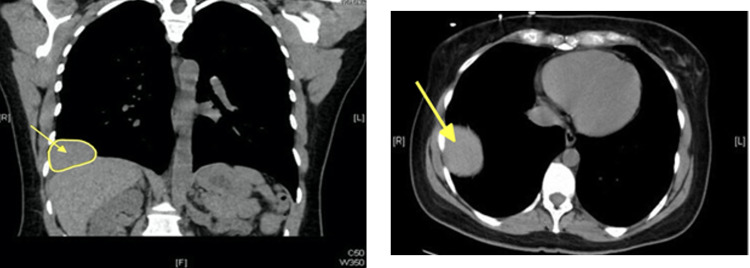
Chest computed tomography axial and coronal views demonstrating right-sided solitary pulmonary mass.
